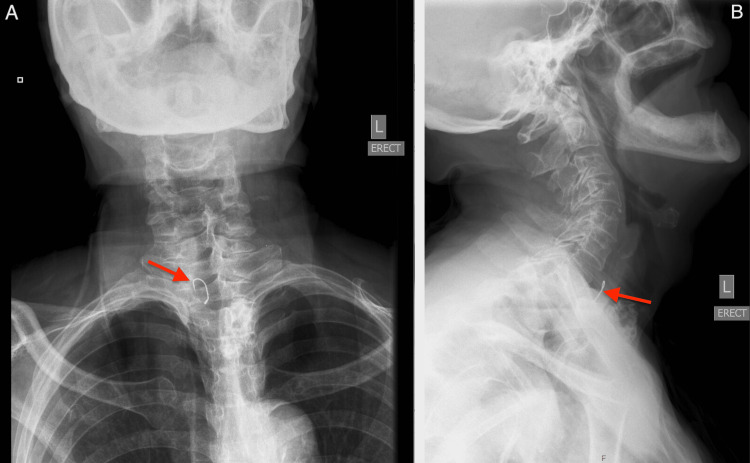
Anterior-posterior (A) and lateral (B) soft tissue neck X-rays demonstrating a bar metallic hook, consistent with a fish hook, present in the soft tissues of the cervical esophagus. The airway is patent and lung apices are clear.
